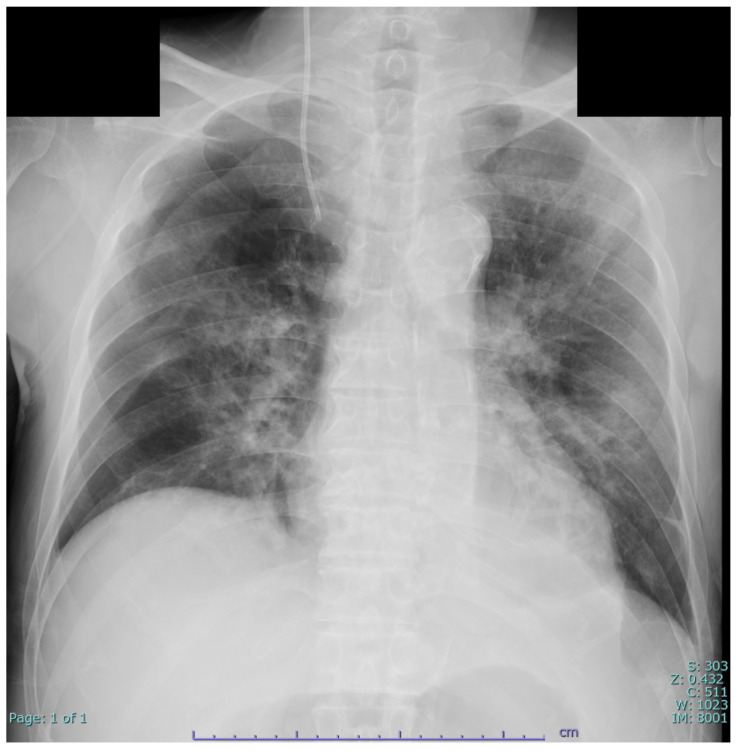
Chest radiograph obtained on day 6. While ground-glass opacities (GGOs) have resolved, reticular shadows persist in bilateral lungs.
